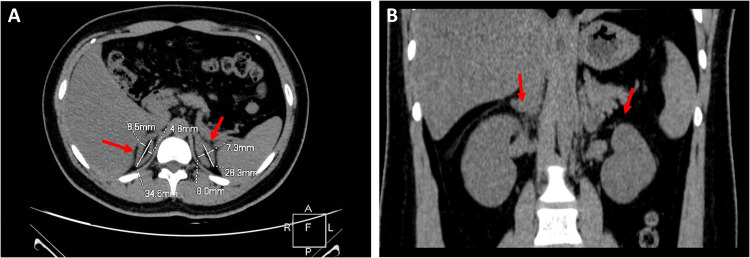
Adrenal computed tomography images. As demonstrated by the transverse (A) and coronal (B) images, the bilateral adrenal volumes in the proband were increased.
